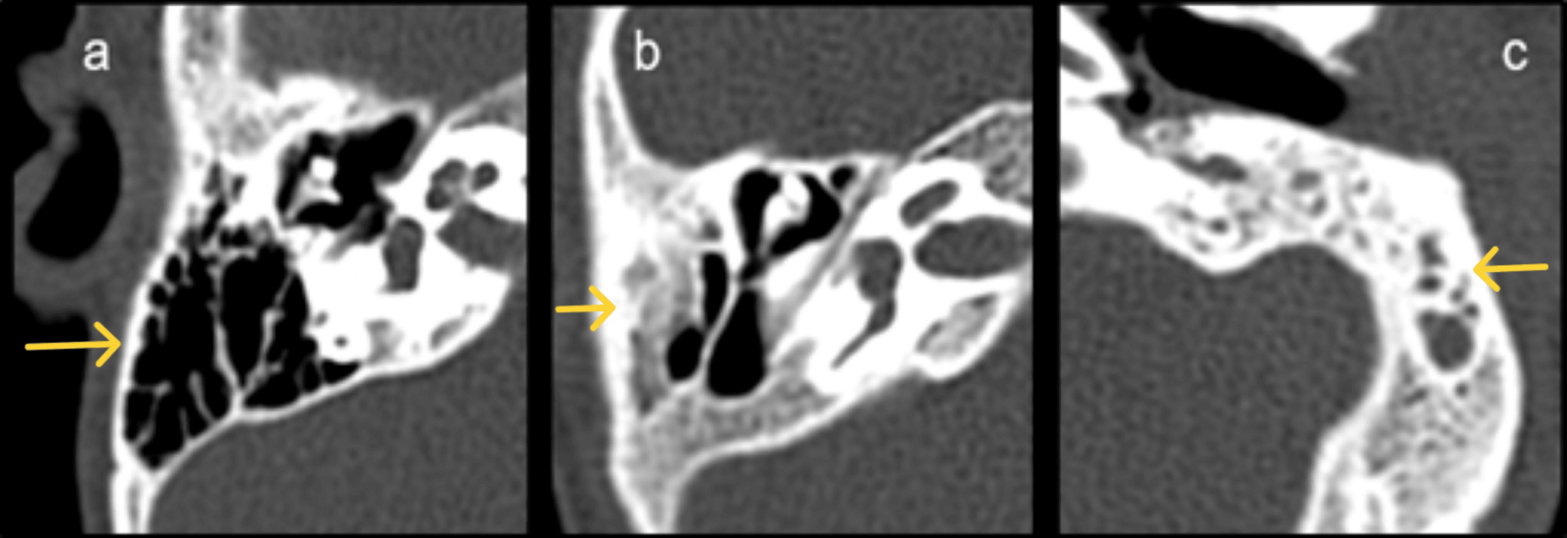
(a)-(c) Axial images of HRCT temporal bone demonstrating mastoid pneumatization pattern. (a) Cellular pattern, (b) diploic pattern, and (c) sclerosed pattern. HRCT: high-resolution computed tomography.
